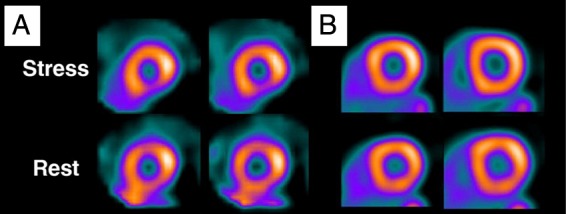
Radionuclide perfusion imaging: (*A*) Hyperaemic stress single photon emission computed tomography and (*B*) positron emission tomography imaging (*B*) of the same patient. (*A*) shows an apparent inferior perfusion defect not present in (*B*) suggesting single photon emission computed tomography attenuation artefact.^50^
